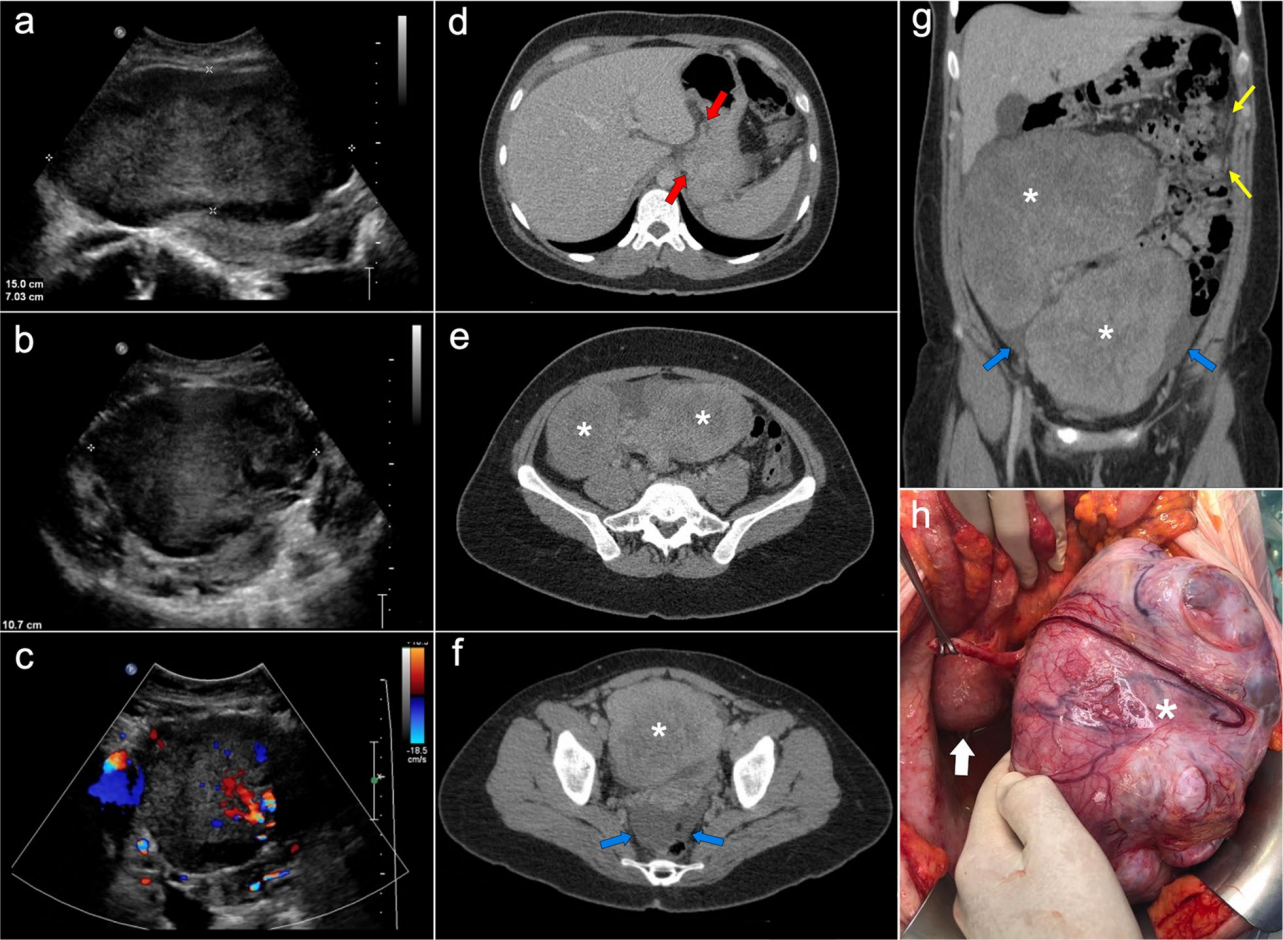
Krukenberg tumors and peritoneal carcinomatosis secondary to a diffuse gastric adenocarcinoma with signet ring cells, in a 32-year-old woman with non-specific abdominal discomfort. First, an abdominal ultrasound was performed, which showed two voluminous solid masses in the lower half of the abdomen (a, b) and demonstrated vascularity within them by color Doppler (c). Axial (d–f) and coronal (g) CT images with intravenous contrast in the portal phase confirmed the presence of two large solid masses of probable adnexal origin (white asterisks), associated with ascitic fluid (blue arrows) and stranding of the omental fat (yellow arrows) suspicious of peritoneal carcinomatosis. Note also the presence of an ill-defined thickening of the gastric wall in the subcardial region, with extension toward the gastrohepatic ligament, suggestive of a primary gastric malignancy (d, red arrows). Subsequently, gastroscopy with biopsy was performed, confirming the diagnosis of a gastric adenocarcinoma with signet ring cells. Palliative debulking surgery with bilateral oophorectomy was decided after multidisciplinary discussion of the case. h Intraoperative view of the pelvis shows the left adnexal mass (white asterisk) and the inferior pole of the right adnexal mass (white arrow), which extends superiorly toward the upper abdomen
